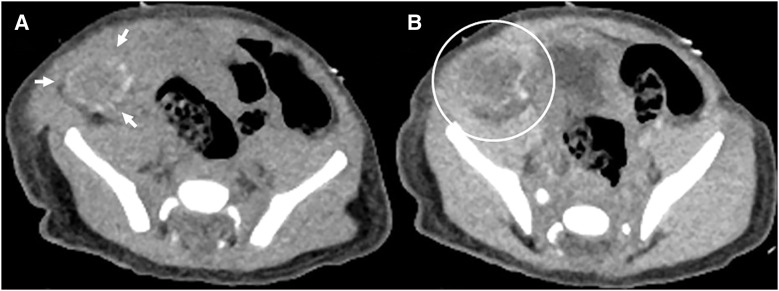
CT findings. (A) Plain CT showing a low-density mass with annular calcifications at the margins (arrows). (B) Contrast-enhanced CT showing no contrast enhancement in the mass (circle).
